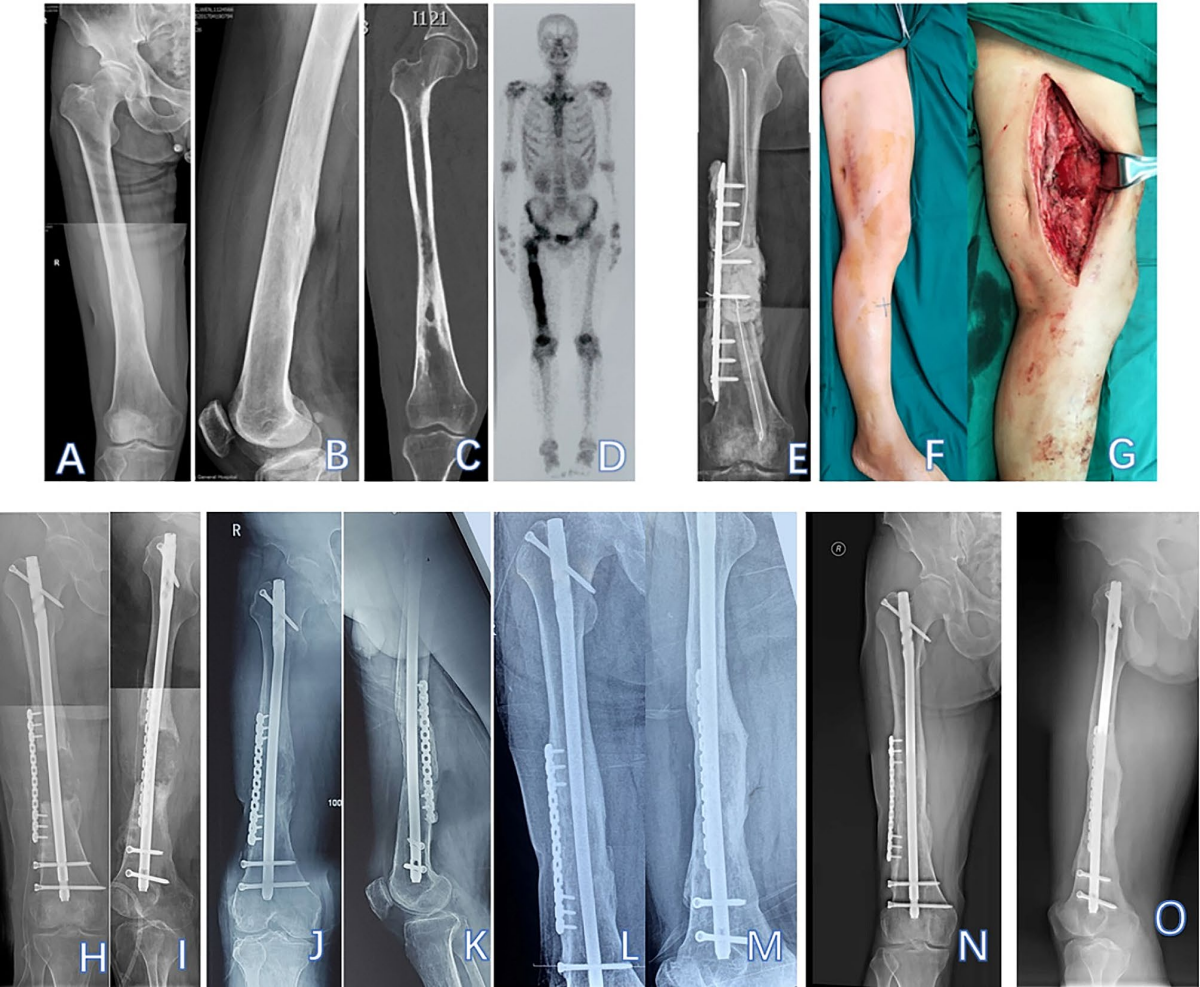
Patient #3, a 56-year-old man with Cinery–Mader type IV chronic haematogenous osteomyelitis in the shaft of the right femur. A: Pre-operative anteroposterior (AP) radiograph of the right femur; B: Pre-operative lateral X-ray of the right femur; C: Pre-operative CT shows bone destruction; D: Pre-operative bone radionuclide scintigraphy; E: Radiograph shows that the lesion was segmentally resected, the bone defect was filled with antibiotic-loaded bone cement, and a cement-coated locking plate was used as temporary stabilisation at T1; F: Wound healing before T2 surgery; G: After the bone cement was removed, an intact induced membrane could be observed. H & I: Radiograph shows an IMN and a locking plate that were used as definitive fixation after T2 surgery; J & K: Callus growth was observed after 5 months; L & M: AP and lateral radiographs show bone union after 30 months; N & O: Radiographs after 52 months
